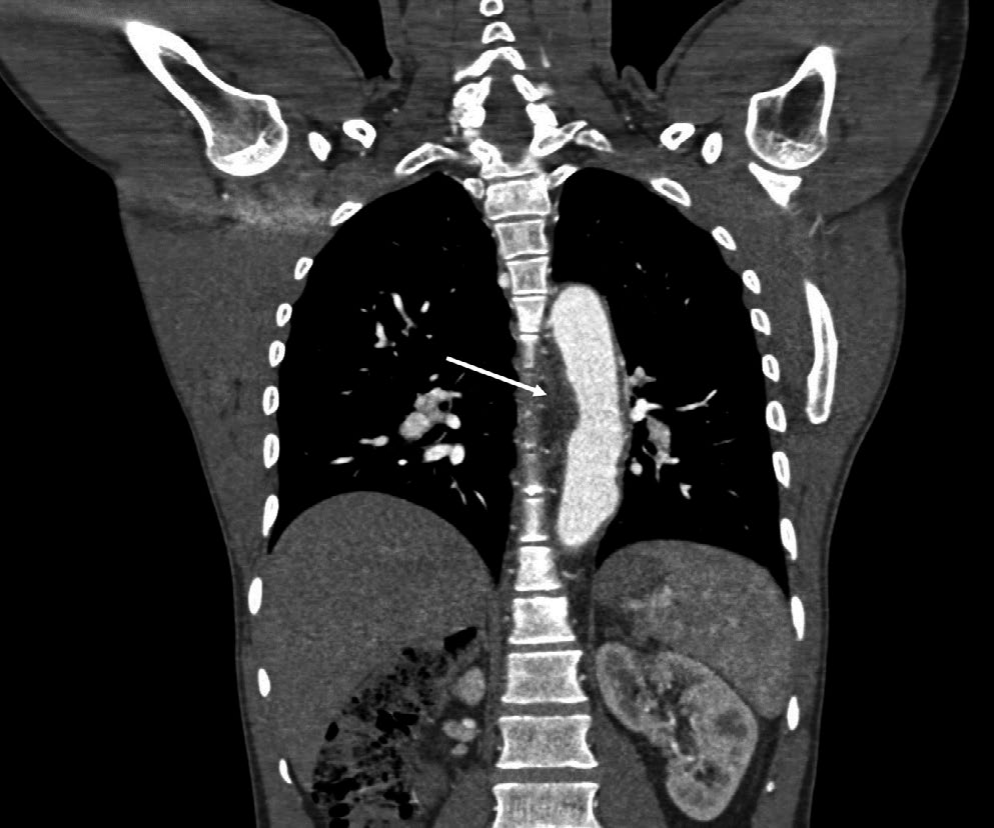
CT aortic angiography coronal reformatted image showing diffuse irregular thickening of the thoracic aorta (white arrow)
